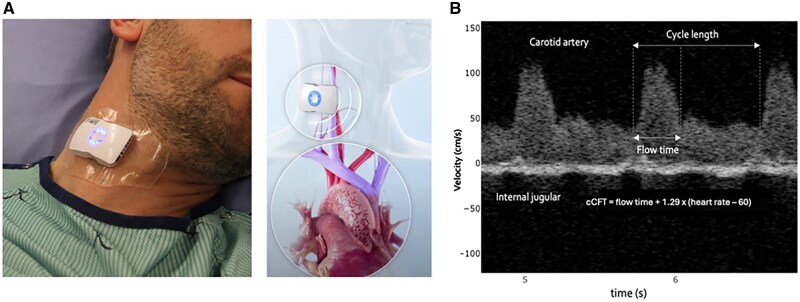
(A) The wireless Doppler ultrasound in place on a patient’s neck. The device is placed over the common carotid artery and internal jugular vein and communicates wirelessly with a nearby tablet. (B) Example of carotid Doppler spectrogram as it appears in the graphical user interface. Features from the device used to calculate ccFT using Wodey’s equation and labeled in white.
